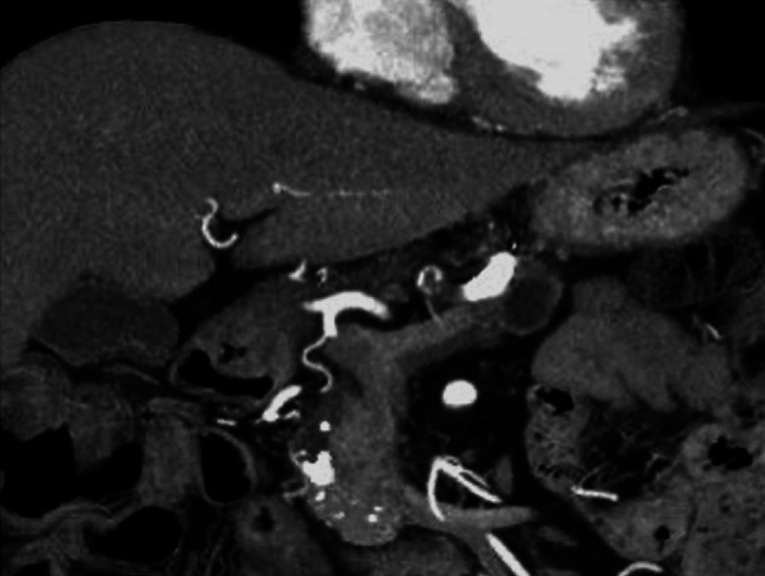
Computed tomography scan showing a stone in the pancreatic head, along with main pancreatic duct dilatation.
